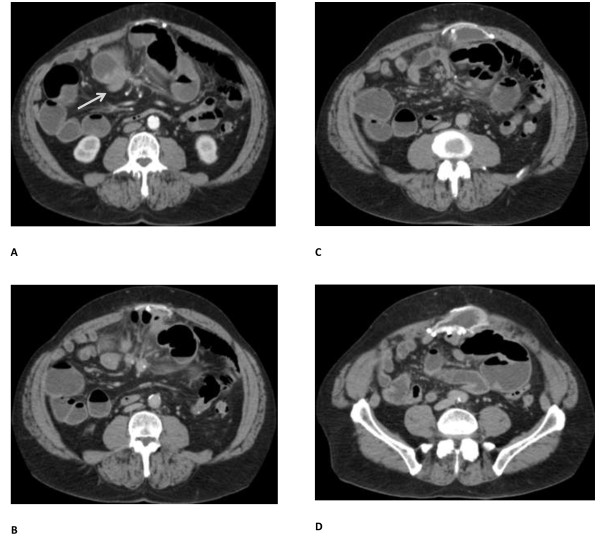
Cross-sectional computed tomography images of patient pre-biologic graft placement. A. Arrow indicates the carcinoid tumor. Presence of synthetic mesh is also shown. B-D. Additional caudad images demonstrating the presence of three synthetic meshes, mesh-associated seroma, and hernia recurrence due to separation of native fascia and mesh interface.
